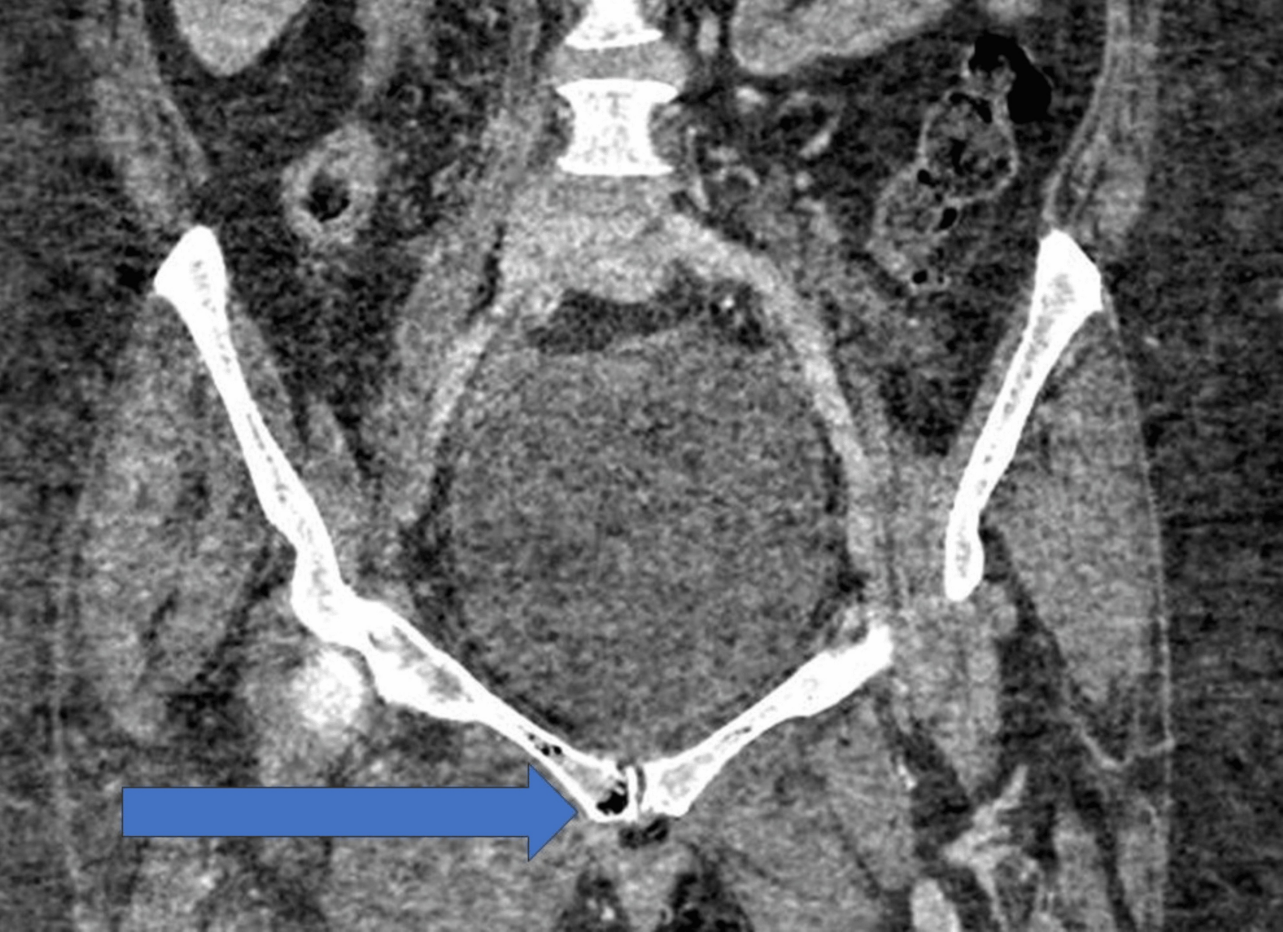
Axial CT image of the pelvis showing air in the pubic bones (emphysematous osteomyelitis) at admission Emphysematous osteomyelitis seen with intraosseous and intramuscular gas surrounding the pubic symphysis, consistent with gas‑forming infection.
